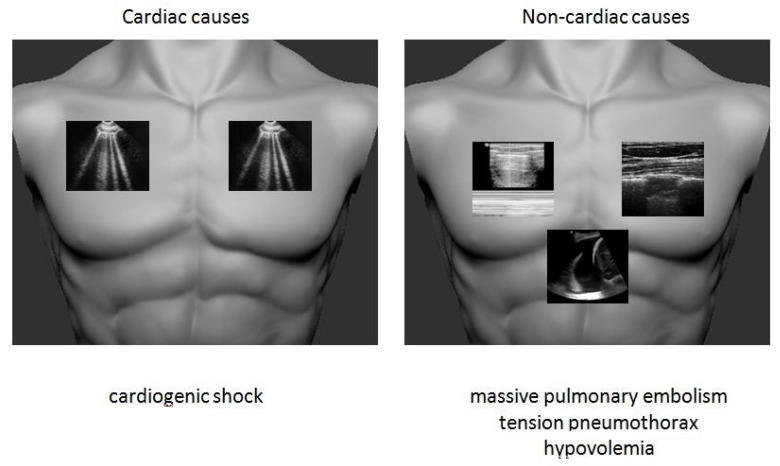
Lung sonographic approach in cardiac arrest.
